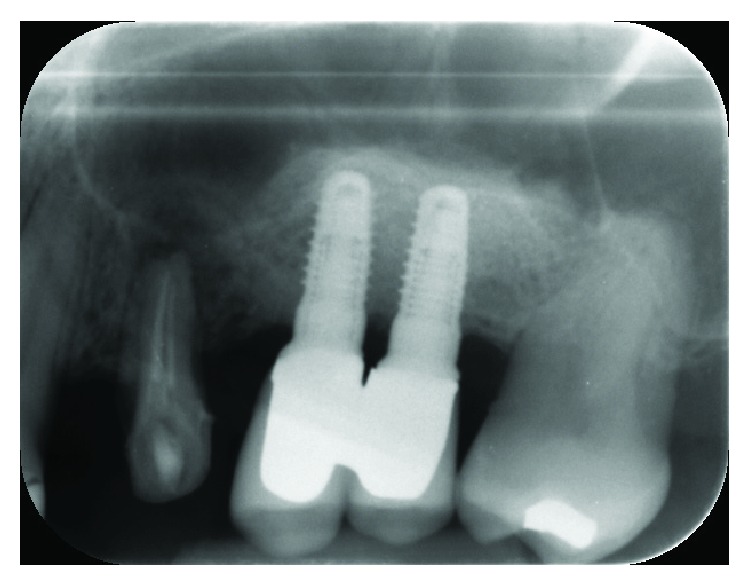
Intraoral radiograph taken after 3 years.
